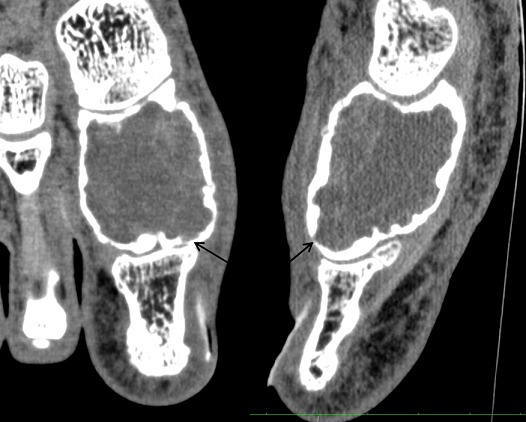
Frontal and sagittal CT view in soft tissue algorithm: expanding osteolytic lesion with focal cortical thinning and without interruption or fluid fluid levels
